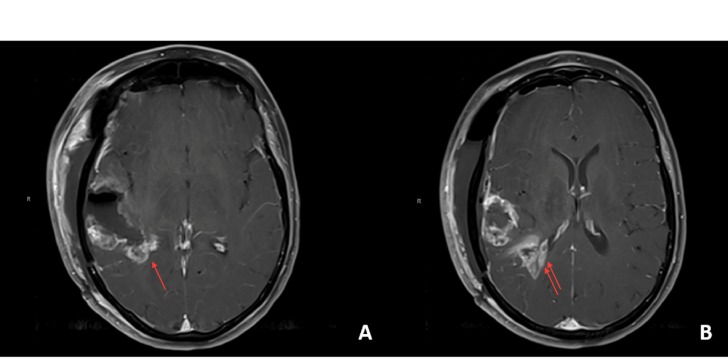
Postoperative magnetic resonance imaging (MRI) A) shows axial T1 MRI with contrast showing expected residual tumor along the Sylvian fissure (arrow) and B) in the posteromedial white matter adjacent to the atrium (double arrows).
